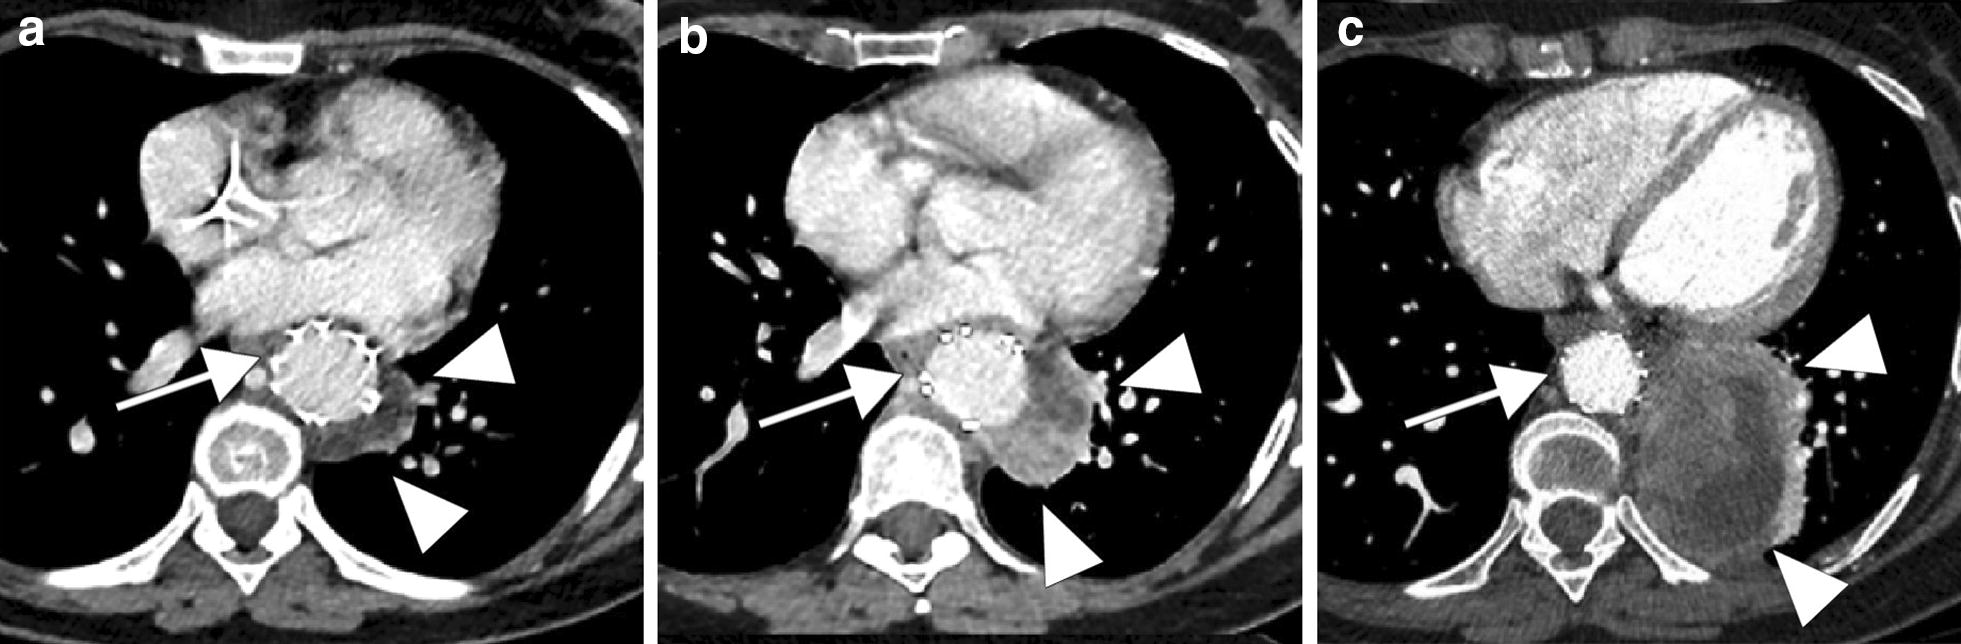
Follow-up CT scans shows rapid progression of the lateral aortic metastasis (white head arrows) near the endoprosthesis (white arrows). a Initial postoperative CT scan shows a non-enhancing lateral aortic lesion which is difficult to differentiate from an excluded aneurysmal cavity. b One month later CT scan shows an important tumor’s size increase with a minimal peripheral enhancement (b). c Six months later, CT scan shows an important tumor progression
